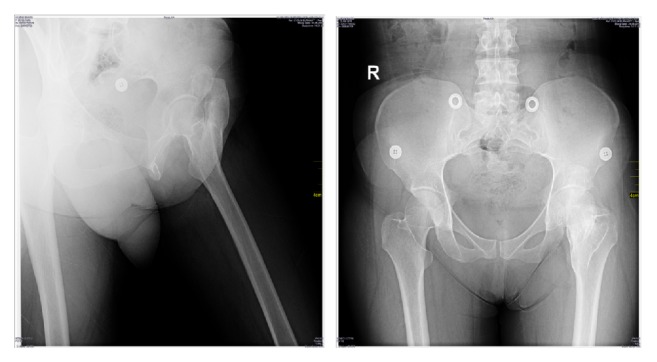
Preoperative radiographic evaluation with X-ray.
